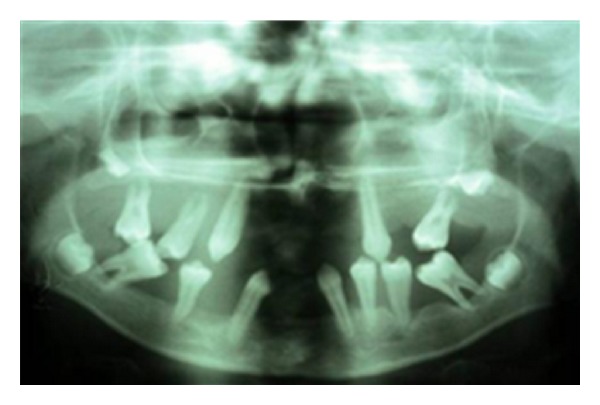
Panoramic radiograph showing “floating teeth appearance.”
